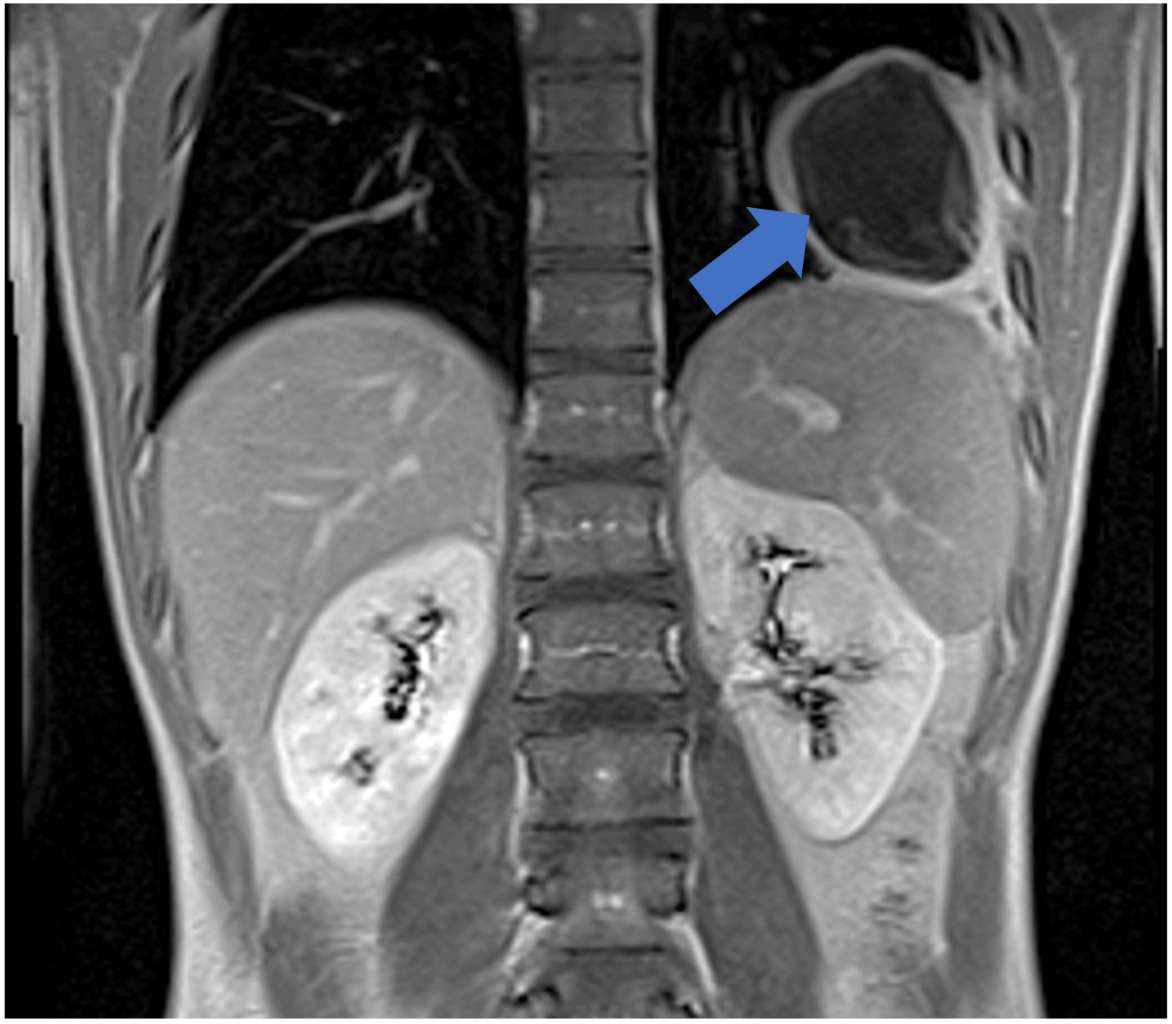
Thoracic and abdominal MRI (coronal view). The blue arrow shows a hydatid cyst in the left lung parenchyma. MRI, magnetic resonance imaging
